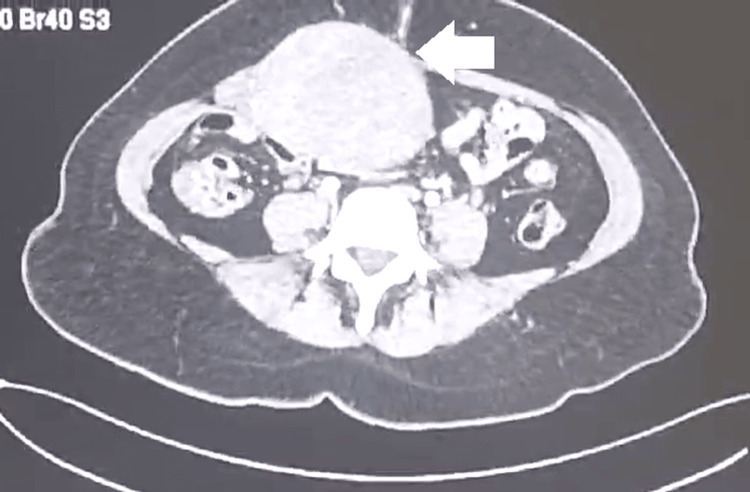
Axial CT image during the arterial phase The scan reveals a well-defined soft tissue mass located in the anterior abdominal wall. The white arrow indicates the central portion of the lesion, showing heterogeneous density and displacement of adjacent structures.
